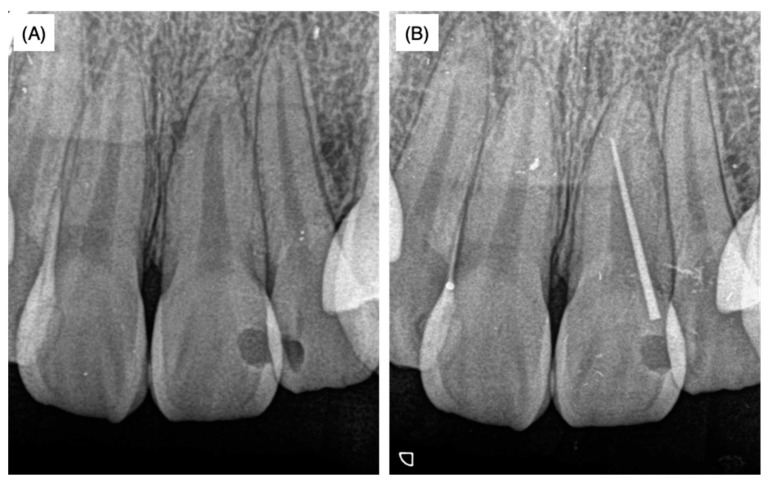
X-ray films without (A) and with (B) the insertion of gutta-percha points when the patient was 18 years and 8 months old. These X-rays were taken at a private dental clinic before the patient came to our university dental hospital.
